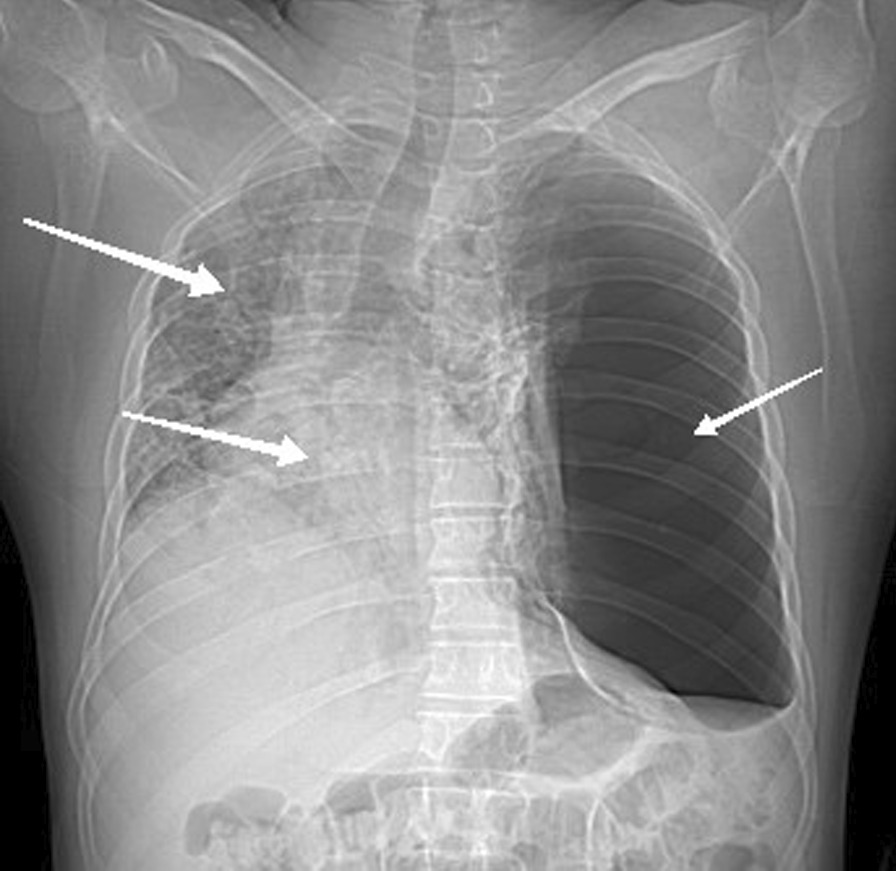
Chest CT scan without contrast injection: the chest X-ray at the patient's bed showed an alveolar syndrome of the right lung (arrow: right/up) with right deviation of the mediastinum (arrow: right/down) and clarity of the right lung except at the apex (arrow: left)
